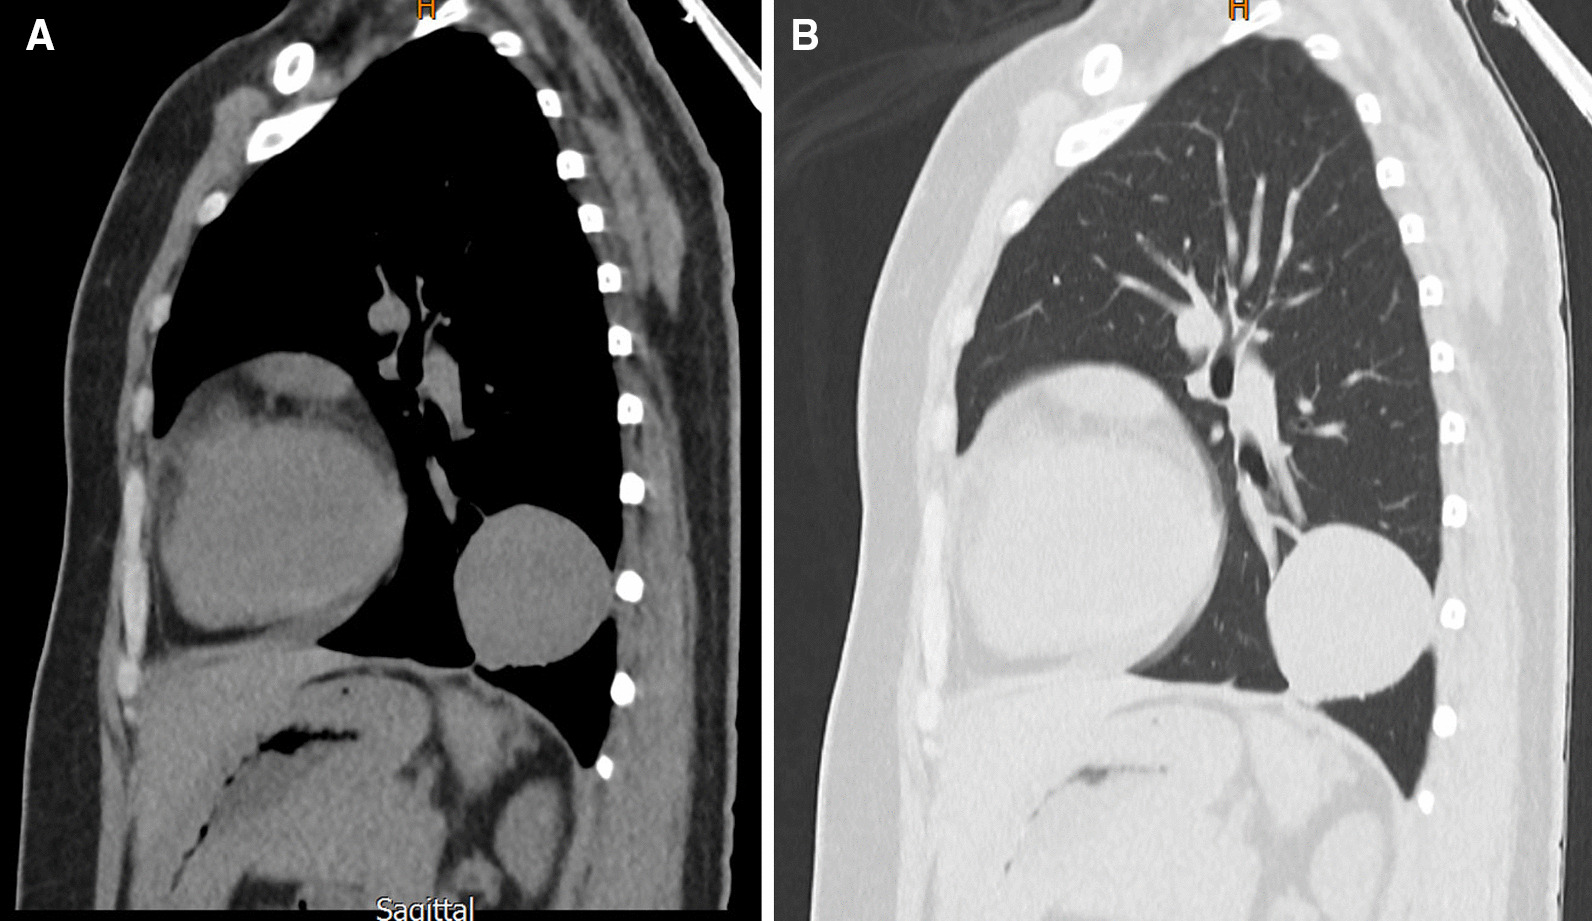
Chest CT scan of the lung mass (lateral view). Two well-defined lung masses are apparent
